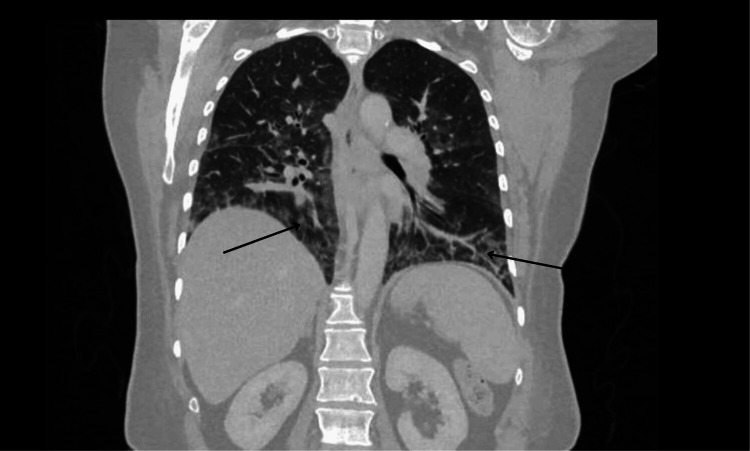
High-resolution CT of the thorax (coronal view) showing bilateral ground-glass changes, reticulations, and inter-lobular septal thickening. Findings are suggestive of interstitial lung disease.
